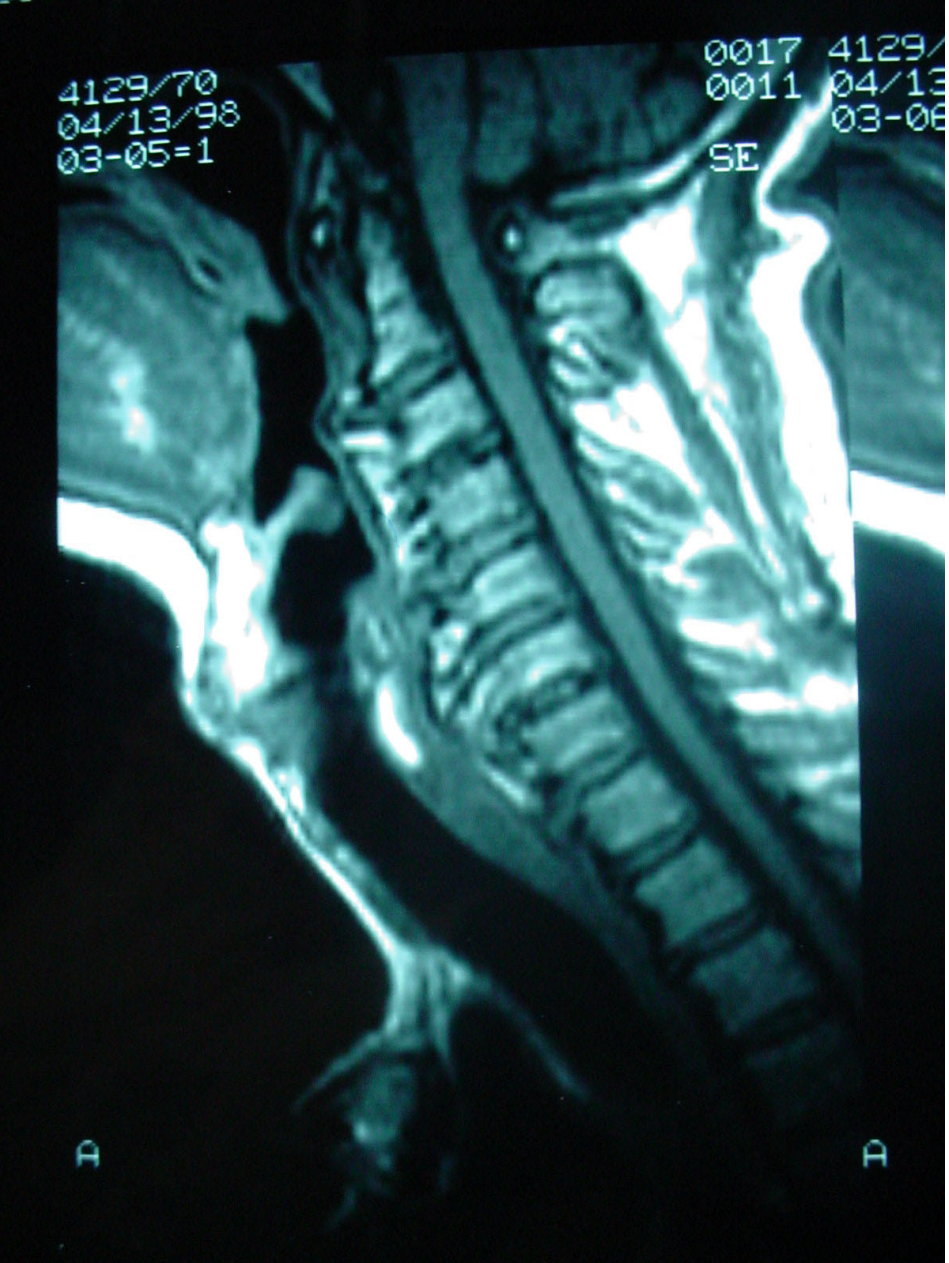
Axial MRI of the cervical spine revealed an elongated ossification of the frontal planes of the vertebral bodies.
